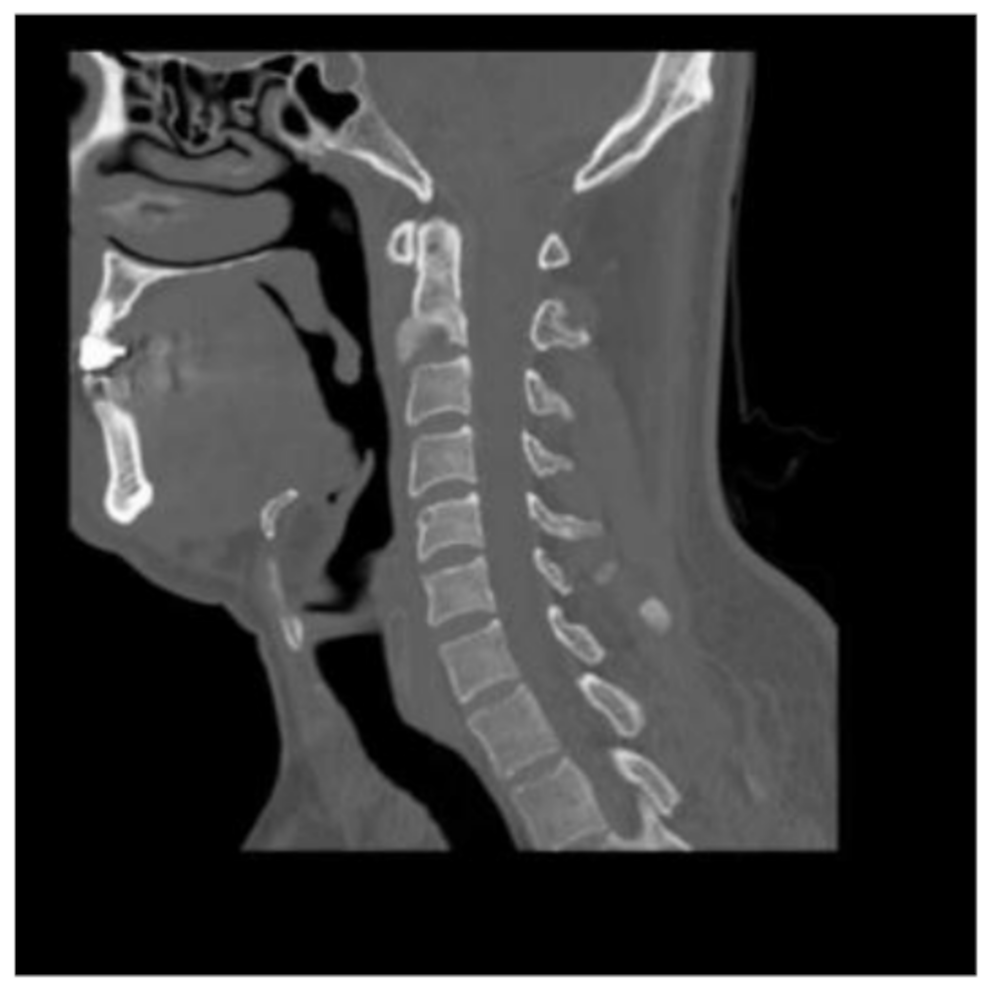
CT scan after 12 weeks showing further progressive consolidation and ventral overgrowth extending to the level of C3
